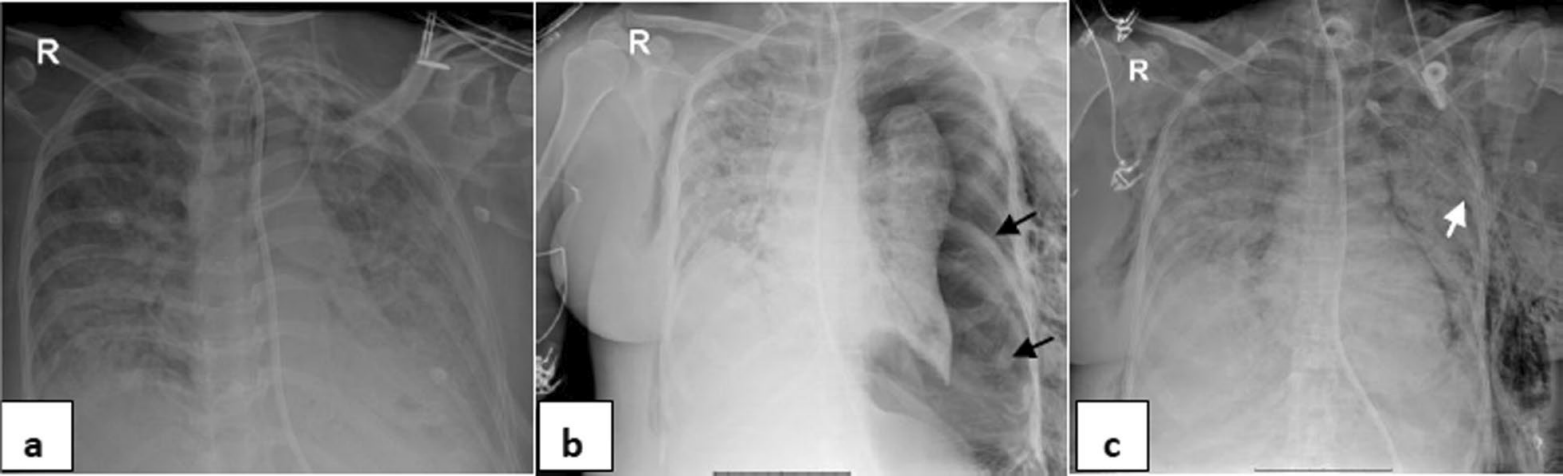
54-year-old female, intubated 12 days post admission. a–c series frontal chest X-ray radiographs demonstrates a large left pneumothorax and surgical emphysema 6 days after intubation (black arrows). There is a left pleural pigtail catheter (white arrow) and re-expansion of the left lung. Note diffuse bilateral ill-defined air space densities throughout both lungs
